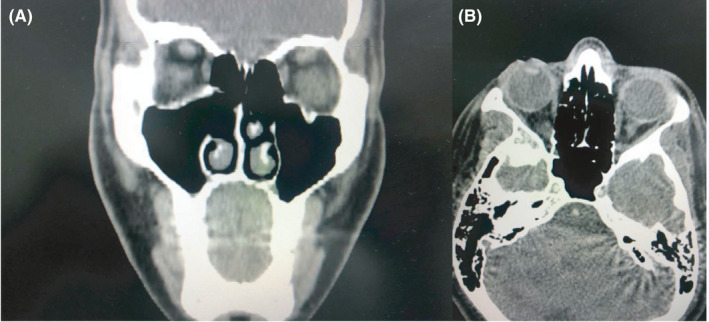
Axial and coronal cuts of CT scan, showing tumor‐free evolution after 9‐month control image
